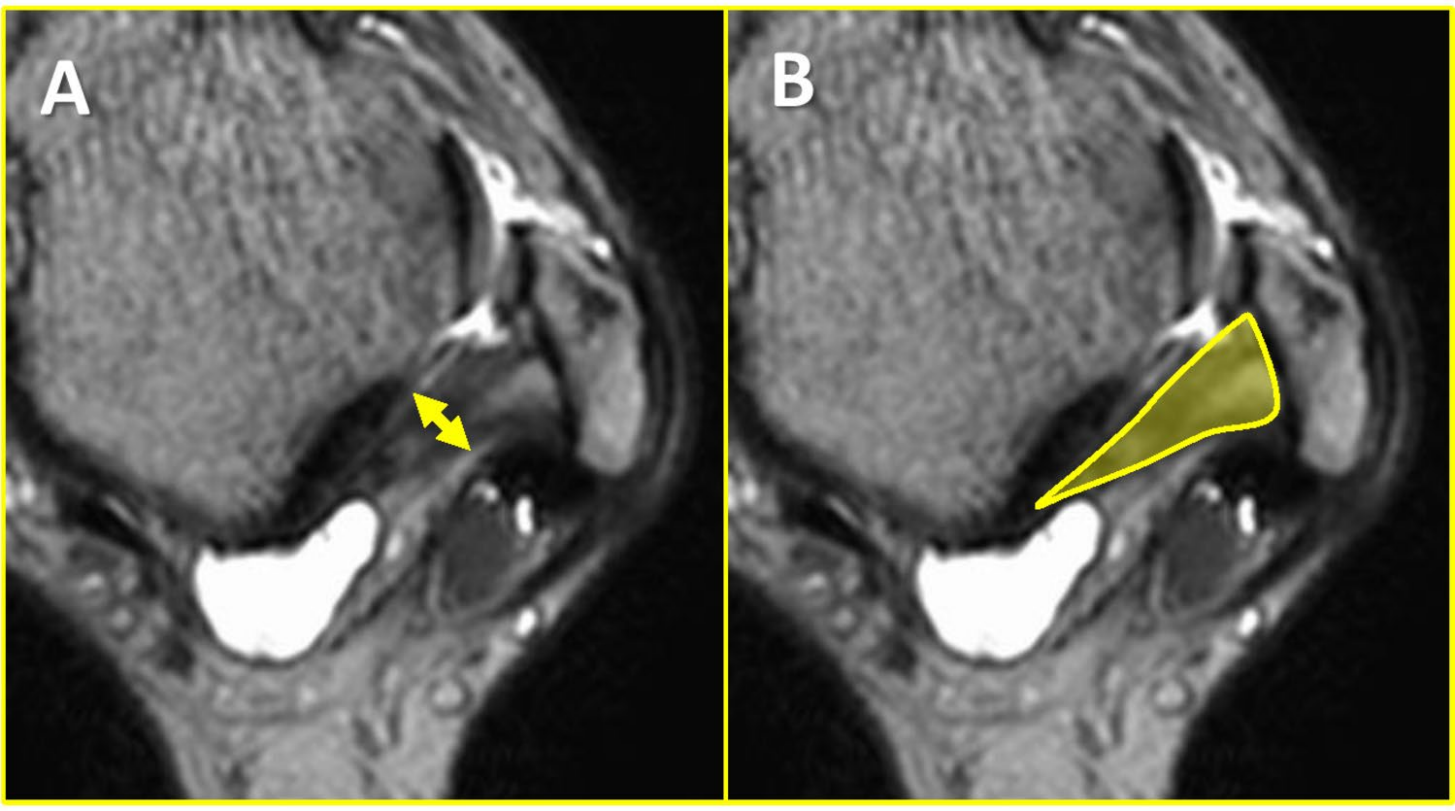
Evaluation of posterior talofibular ligament (PTFL) injury on magnetic resonance imaging (MRI). A Measurement of PTFL thickness (PTFLT) B. Measurement of the PTFL cross-sectional area (PTFLCSA)
